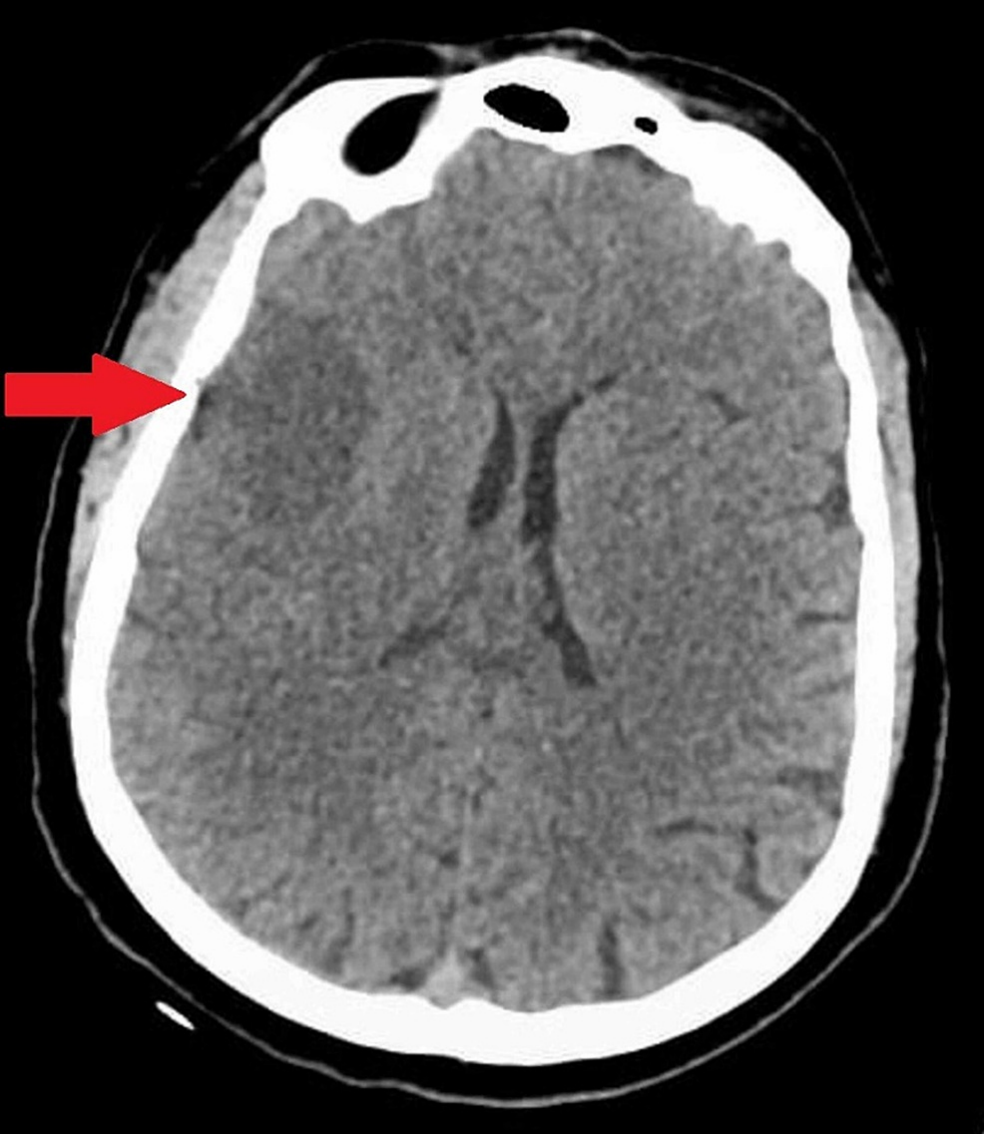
Initial non-contrast CT head on the day of admission. The arrow indicates the ischemic region that is visible as a hypodense fronto-parietal area extending to the insular cortex and external capsule. CT: Computed Tomography
